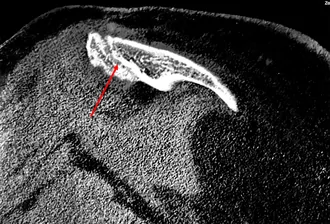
Six‐month postoperative coronal CT image showing bony union at the right acromial fracture site (red arrow).
